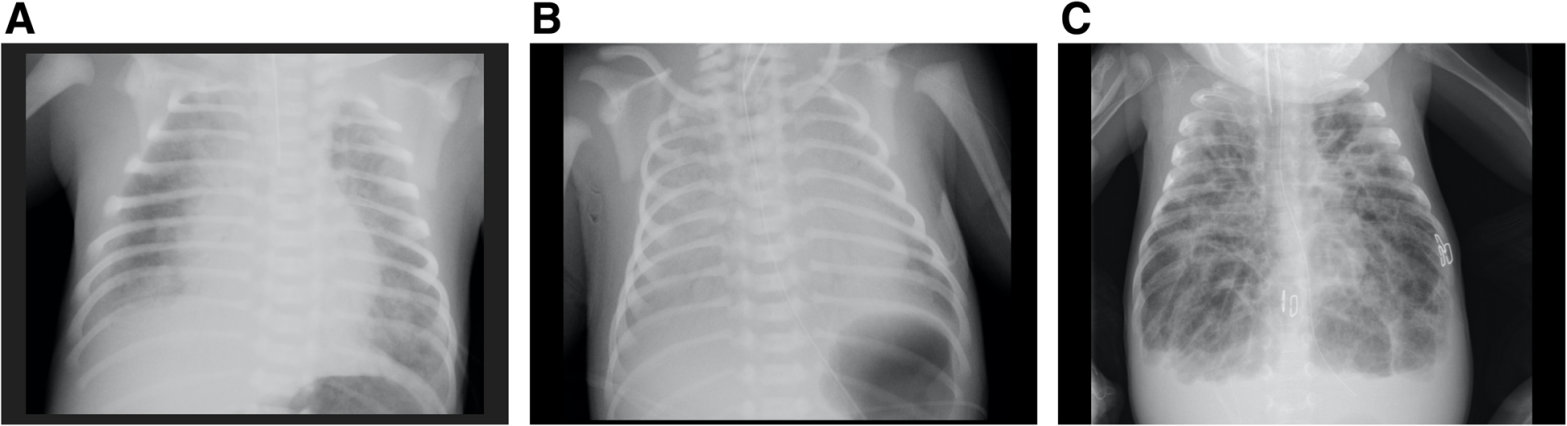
A Chest X-ray at admission. B Chest x-ray on the second day of hospitalization, showing progressive bilateral diffuse opacities. C Chest X-ray at 84 days of age
